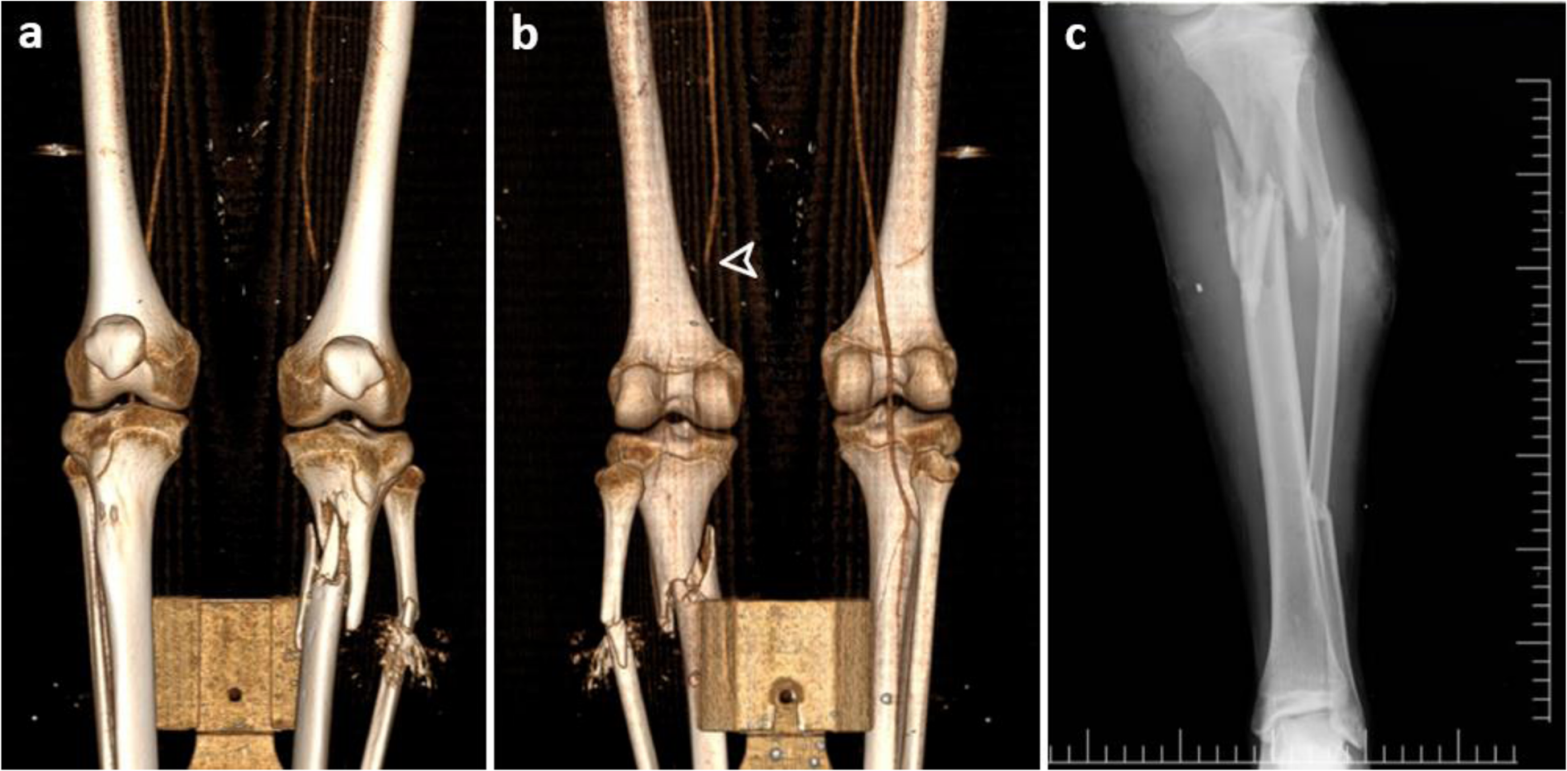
Images of a patient with popliteal artery injury due to open fractures in the tibia and fibula. a-b 3D-reconstructed computed tomography angiography images in the anterior and posterior views showing type 3C open fractures in the tibia-fibula and no blood flow in the popliteal artery (arrowhead). c Radiography image showing open fractures in the tibia and fibula
